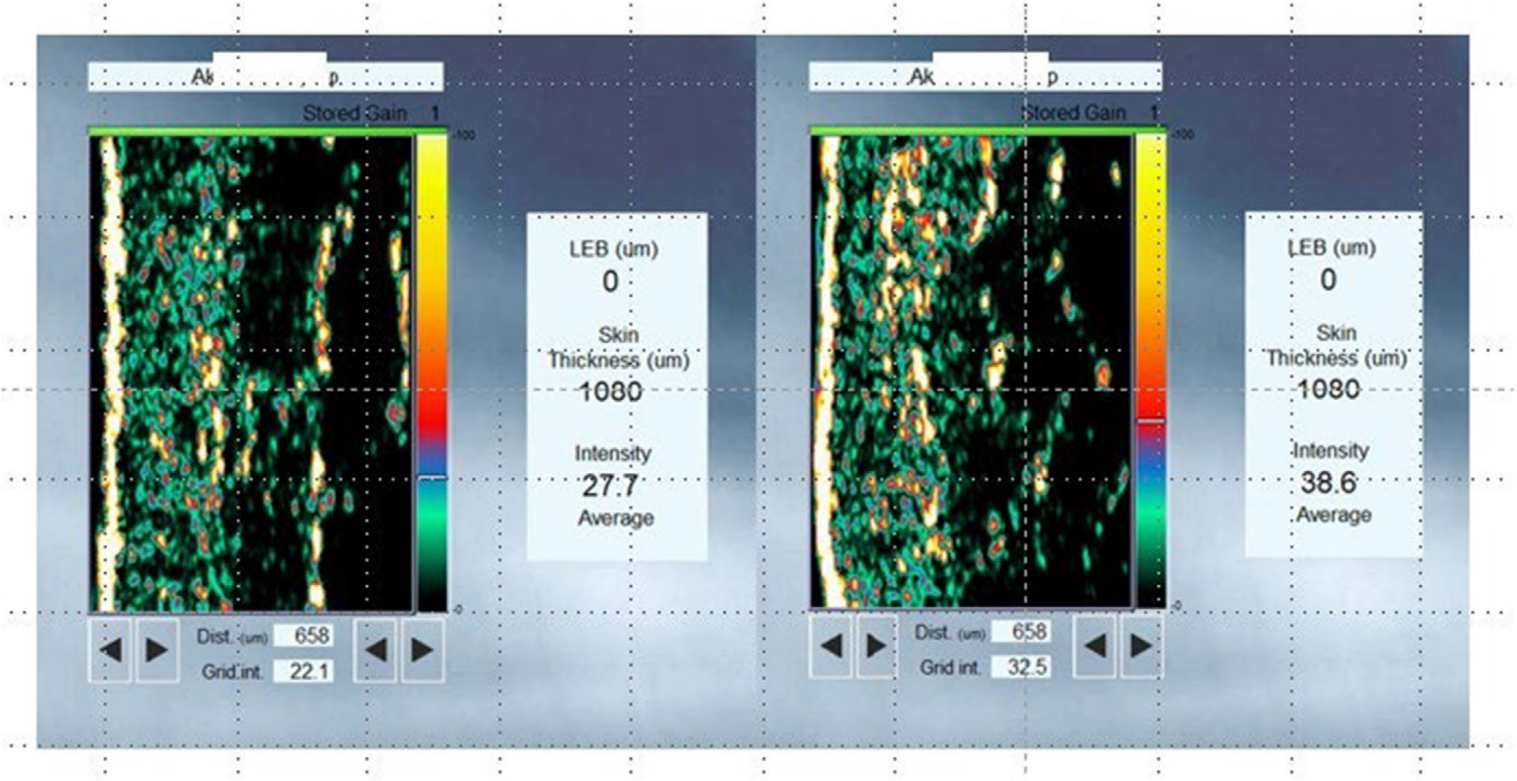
Improvement of intensity of collagen deposition score in dermis and RC assessed with high‐resolution ultrasound.
